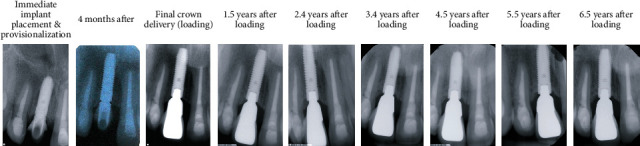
Periapical radiographs taken at immediate implant provisionalization and at various times during the 6.5-year recall period.
